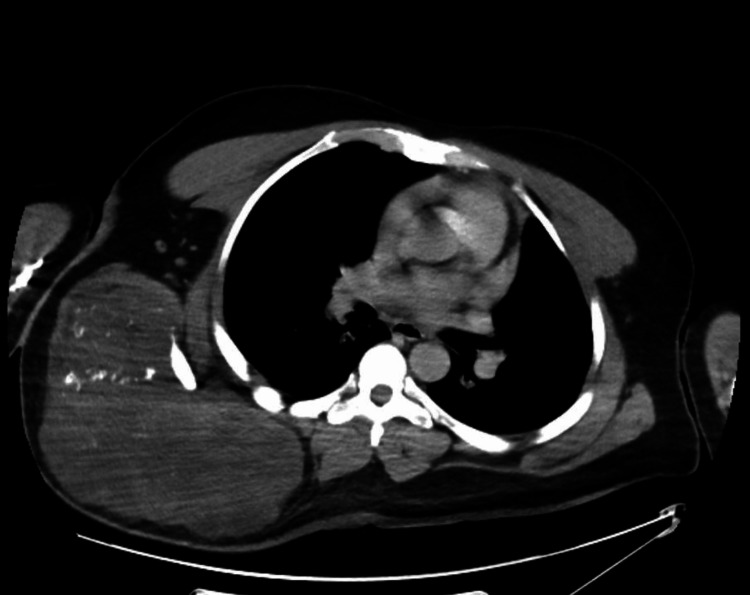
Soft tissue window axial CT view showing a large, aggressive right scapular bone lesion.
